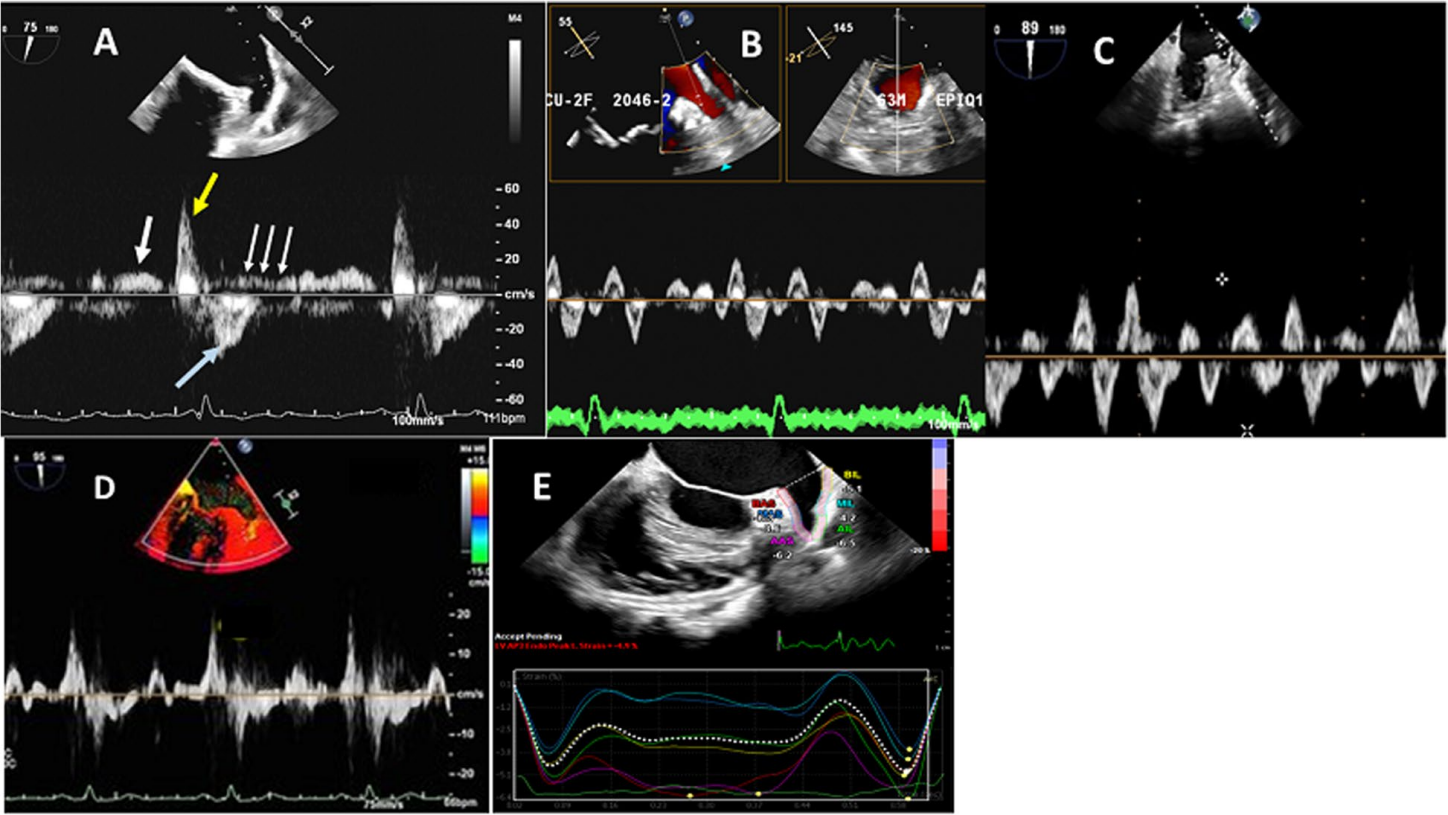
Pulsed wave Doppler of the left atrial appendage (LAA) flow velocity pattern. A In normal sinus rhythm (white thick arrow points toward the early diastolic emptying velocity, yellow thick arrow points toward the late diastolic emptying velocity, blue thick arrow points toward the LAA filling velocity, the three small arrows point toward the systolic reflection waves. B In patients with Atrial fibrillation. C In patients with atrial flutter, the lower panel shows (D) the tissue velocity pattern of the LAA as obtained by tissue-Doppler imaging and (E) longitudinal strain values and curves of the LAA measured by speckle tracking echocardiography
